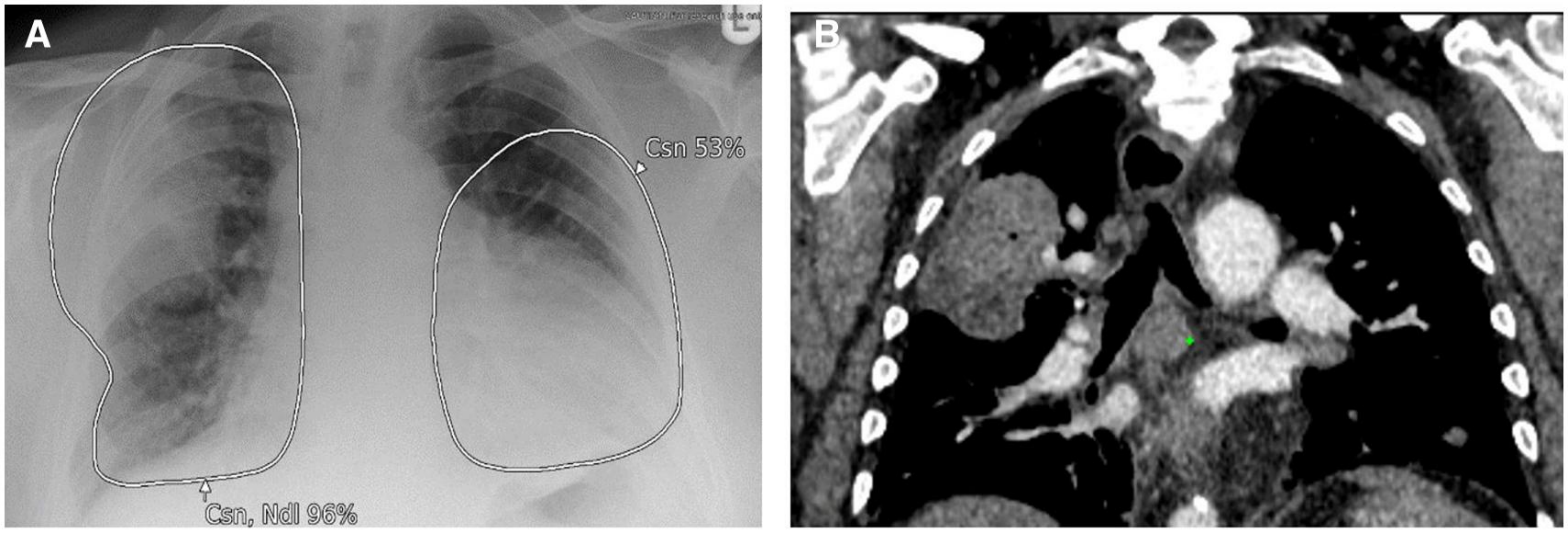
An 84-year-old male, with no history of smoking but with cardiac symptoms, underwent a CXR (A), reported as bilateral consolidation, instigating no further follow-up imaging. The AI software highlights similar suspicion of consolidation but with secondary suspicion of an underlying nodule on the right side (A, largest white circle). One year and 3 months later, the patient attends for a CT scan (B) due to progressive symptoms, which demonstrates a large right lung mass confirmed as stage IV LC. AI = artificial intelligence; CXR = chest radiograph; LC = lung cancer.
